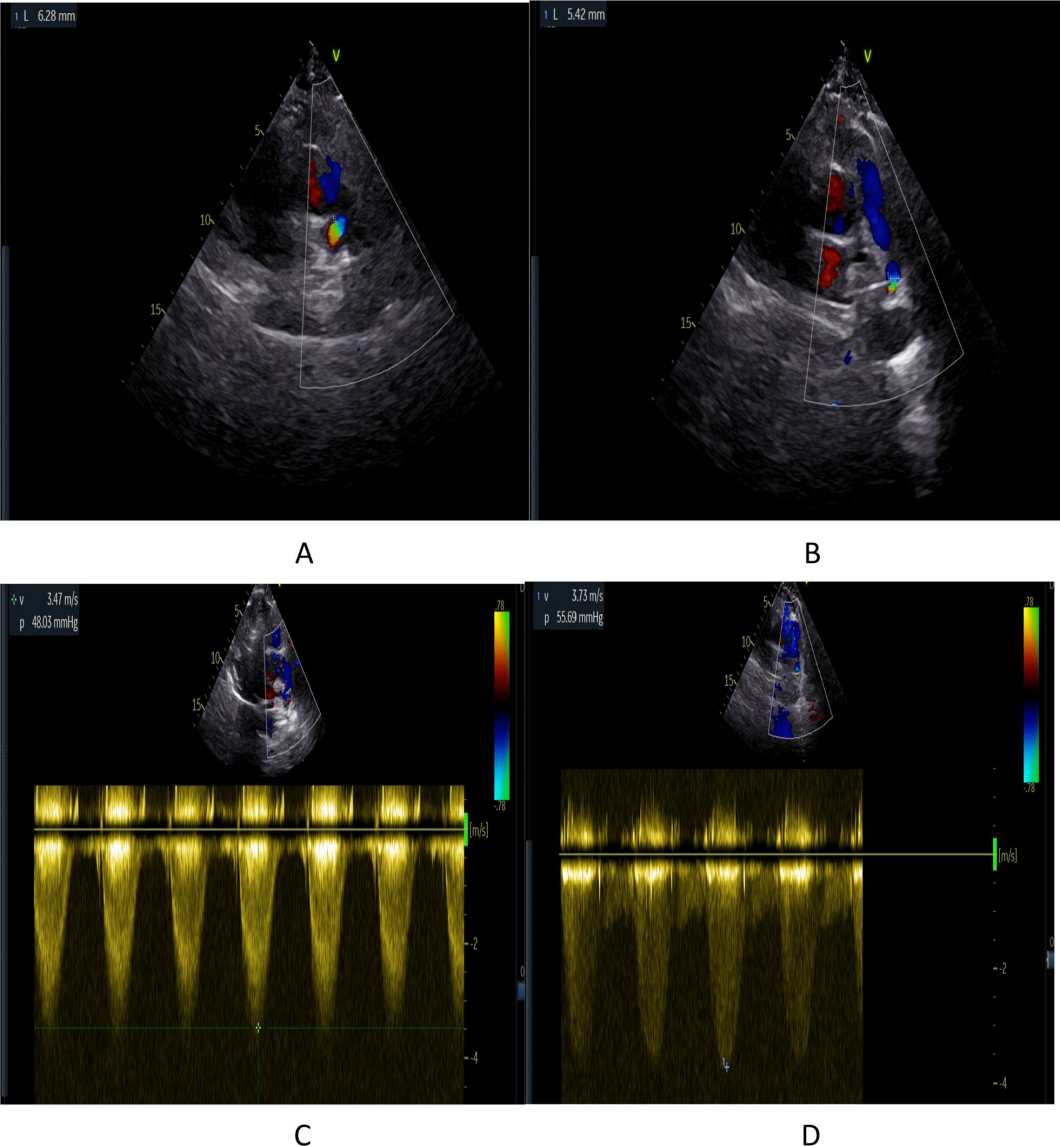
A–D Echocardiogram (A), B the narrowest diameter of right and left proximal PA. C, D The blood flow velocity and the maximum pressure difference of the right and left PA
